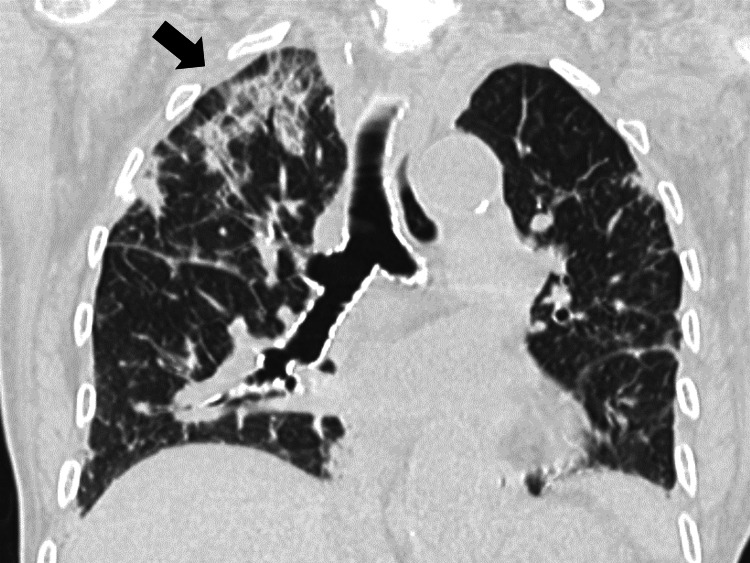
Initial chest computed tomography showing bilateral pulmonary infiltrates predominantly on the right lung
